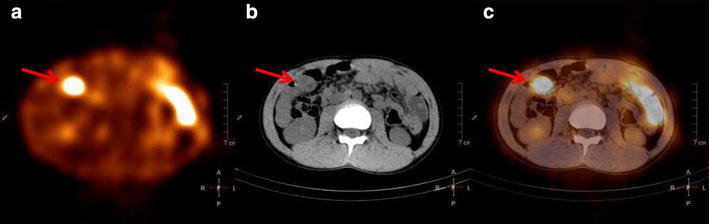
Axial SPECT/CT image of the Meckel’s diverticulum. a Axial image SPECT shows one focal concentration in the right abdomen (*arrow*). b CT demonstrated a blind-ending tubular structure in the right abdomen (*arrow*). Anatomical relationship between the focal concentration and right kidney cannot be identified. c Axial SPECT/CT fusion image showed that it was in front of the kidney that the focal concentration lays (*arrows*)
